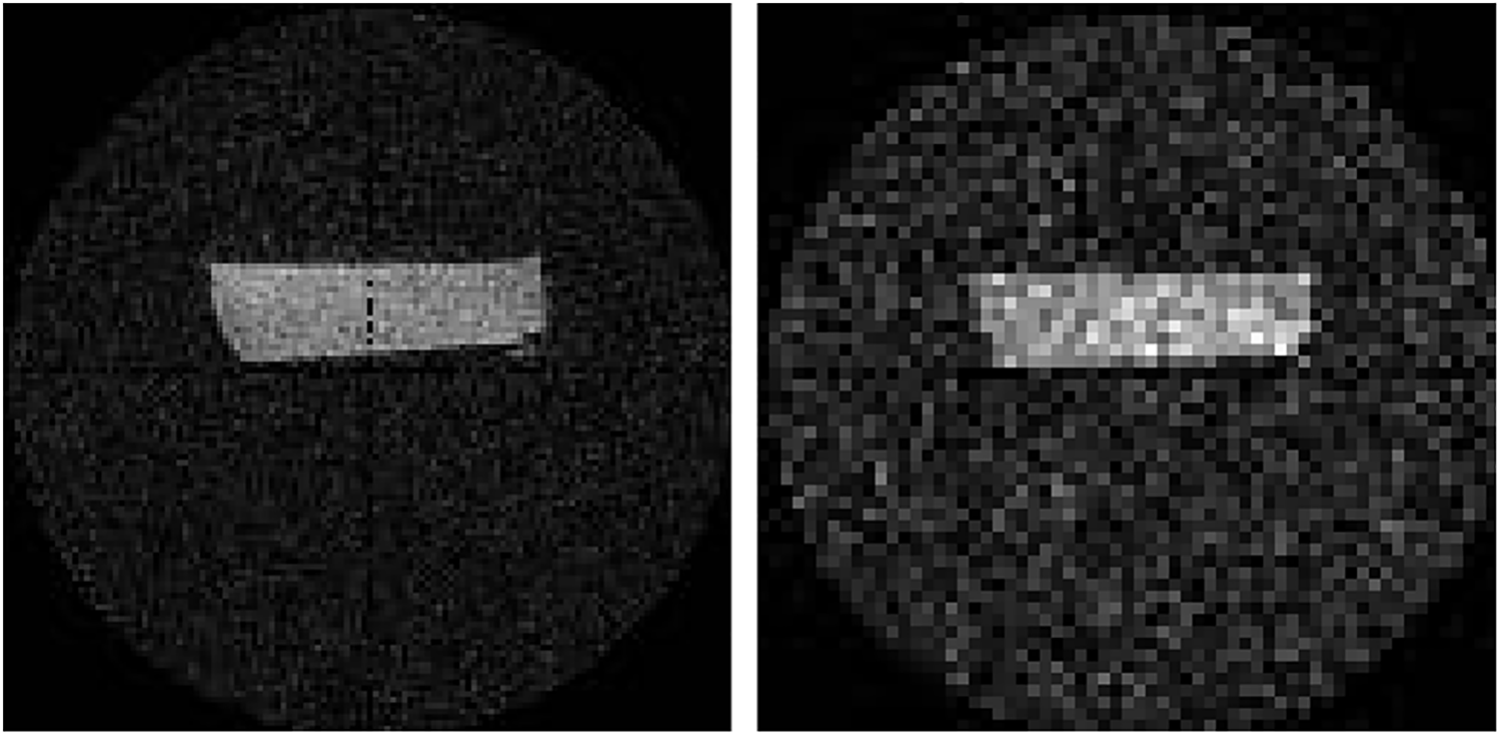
^1^H- and ^23^Na-ZTE MRI of an aqueous model solution containing 100 g NaCl / l acquired with LG_4_ and CB_3_ at 9.4 T.
